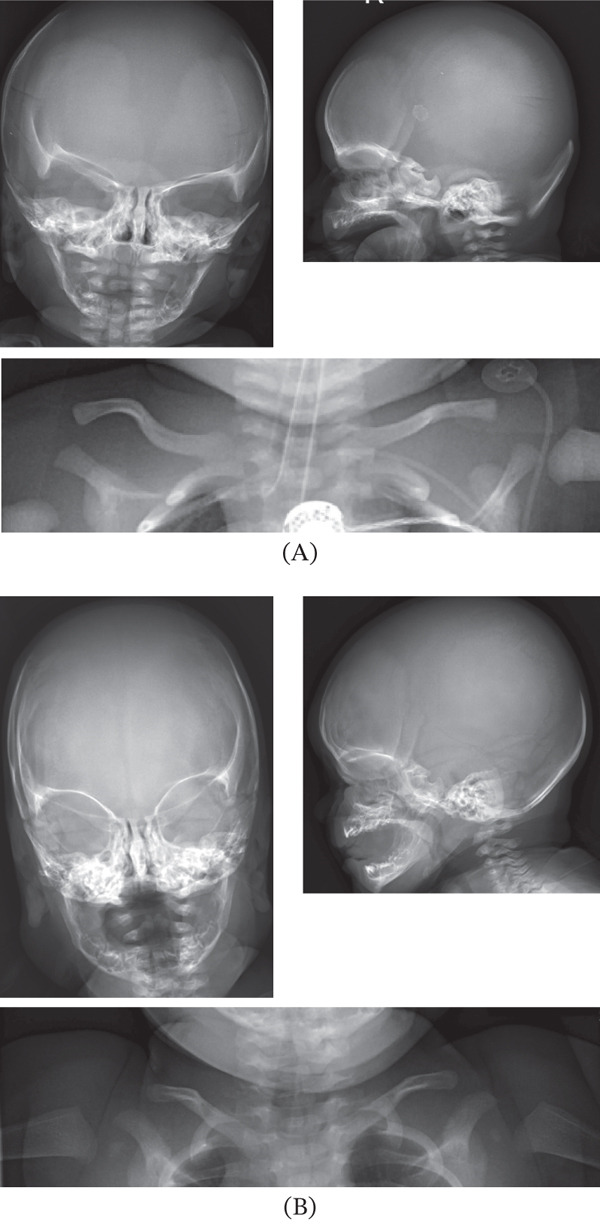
Skull radiographs. (A) Patient 1 (Day 8) (left: AP skull, right: lateral skull, bottom: clavicles): note the wide anterior fontanelle with widely patent sagittal and metopic sutures, with normal clavicles. (B) Patient 2 (2 months) (left: AP skull, right: lateral skull, bottom: clavicles): note the poorly ossified skull, wide anterior fontanelle with wide intraparietal sutures, but normal clavicles.
